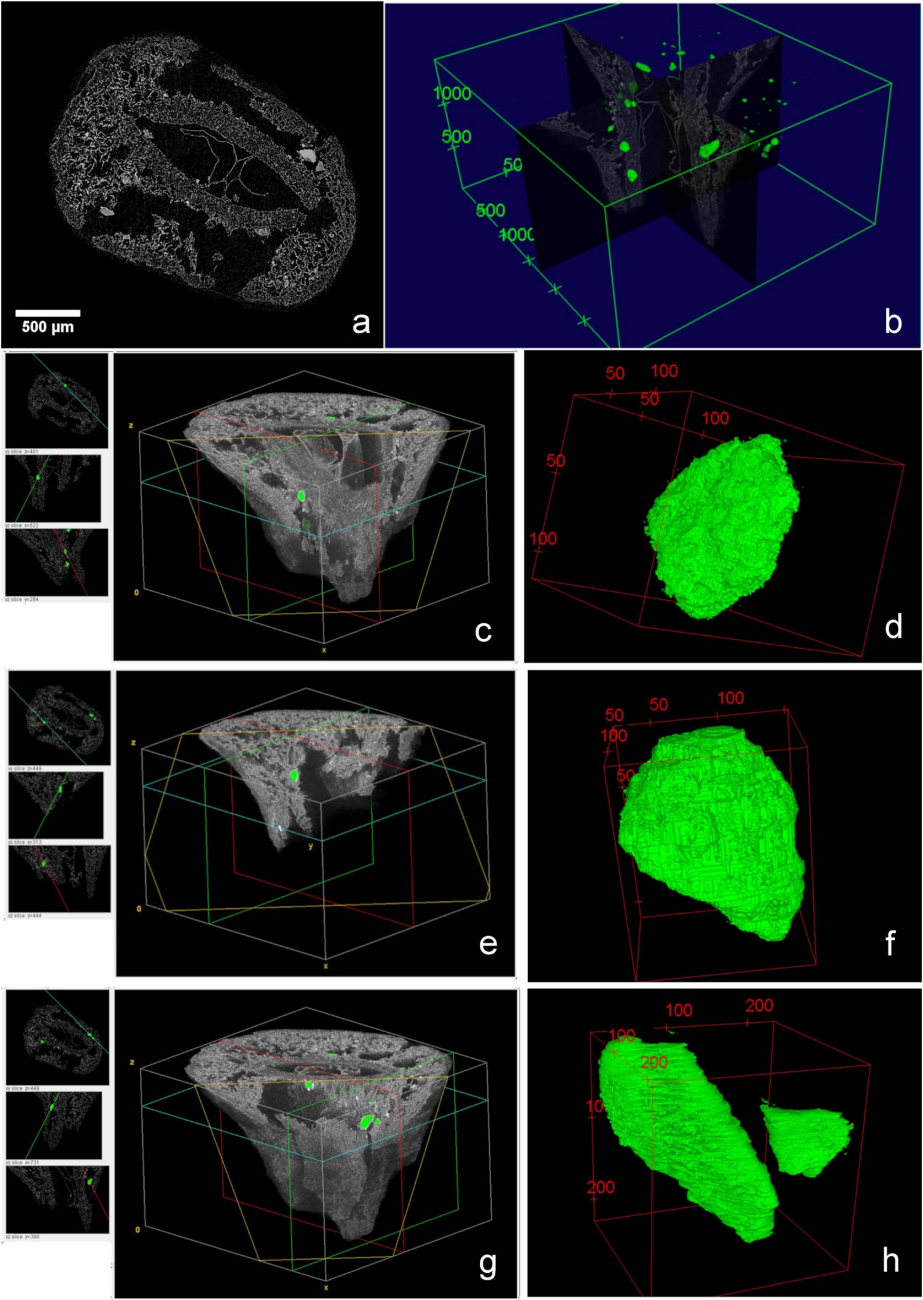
2D Visualization of dense amorphous particles in a virtual transversal section from sample SMC_01 (a) Volume reconstruction of amorphous particles in SMC_01 placed in the orthogonal section of the whole sample volume; (b) Volume reconstruction of the same sample showing the localization of three amorphous particles and their respective 3D image; (c–e) (CT Analyzer; ImageJ-FIJI, plug-ins: 3D Viewer and Volume Viewer).
